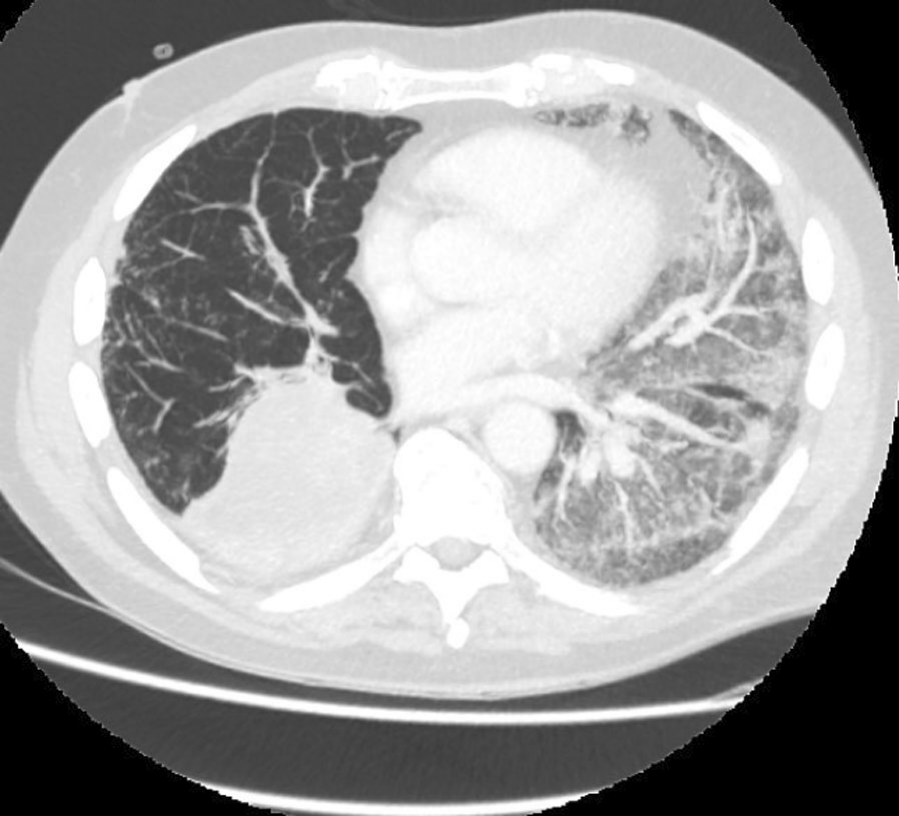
Case 2—CT chest lower lobes with deterioration of pneumonitis despite treatment
